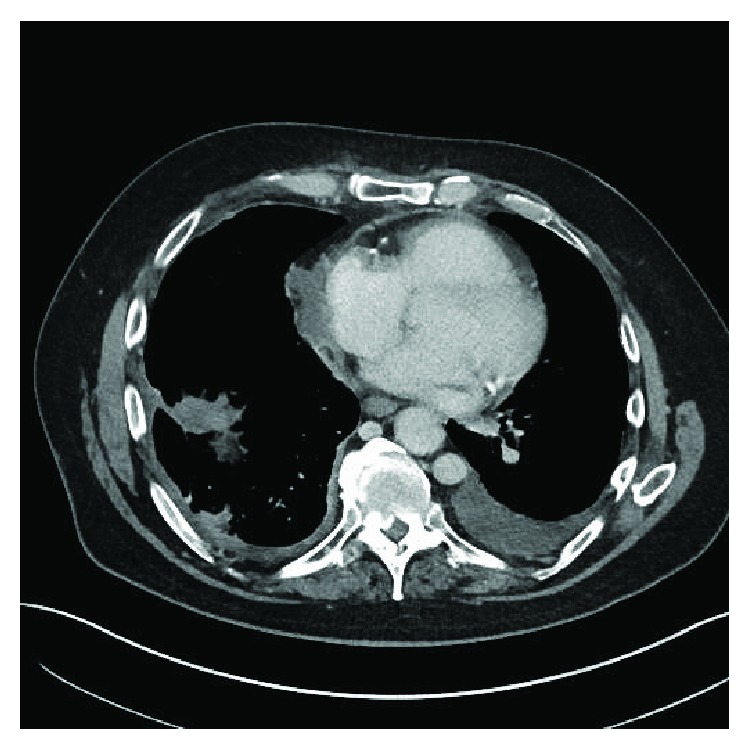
CT scan of the chest obtained one week after the pericardial window showing loculated pericardial fluid adjacent to the right atrium that is slightly higher in attenuation, suggestive of bloody or proteinaceous effusion.
